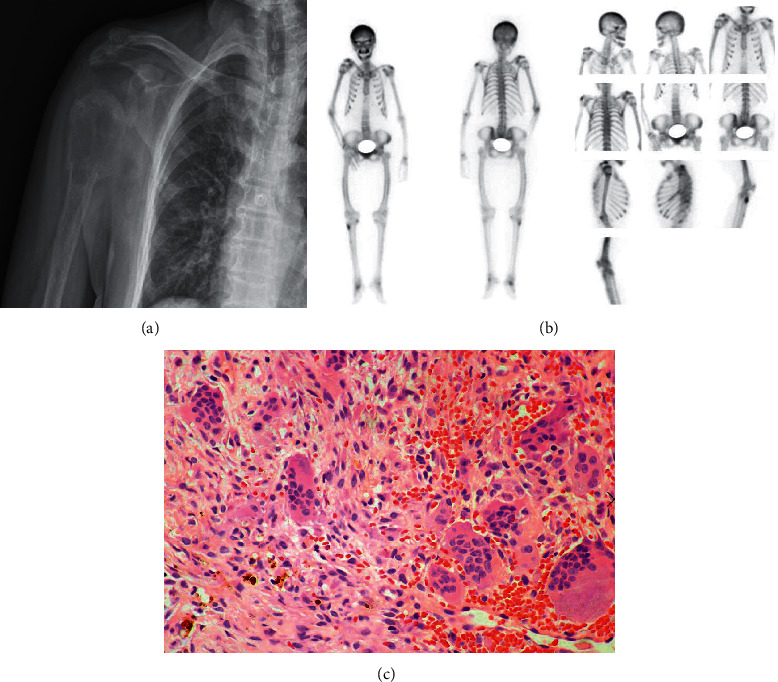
(a) Chestx-ray showing loss of cortical continuity through the surgical neck of the right proximal humerus with a lytic lesion, suggesting an impacted fracture and cortical alteration of the distalclavicle. (b) Bone scintigraphy with MDP-99 m Tc, shows the abnormal distribution of the radiotracer due to diffuse and generalized uptake increase in the bone system, with a reduction in soft tissues. (c) Proximal right humerus biopsy showing multiple giant osteoclast cells, dispersed among a fibrous stroma with hemorrhagic foci and hemosiderin deposits and scarce osteoid with prominent resorption by osteoclasts indicating compatible with Brown tumor due to hyperparathyroidism.
